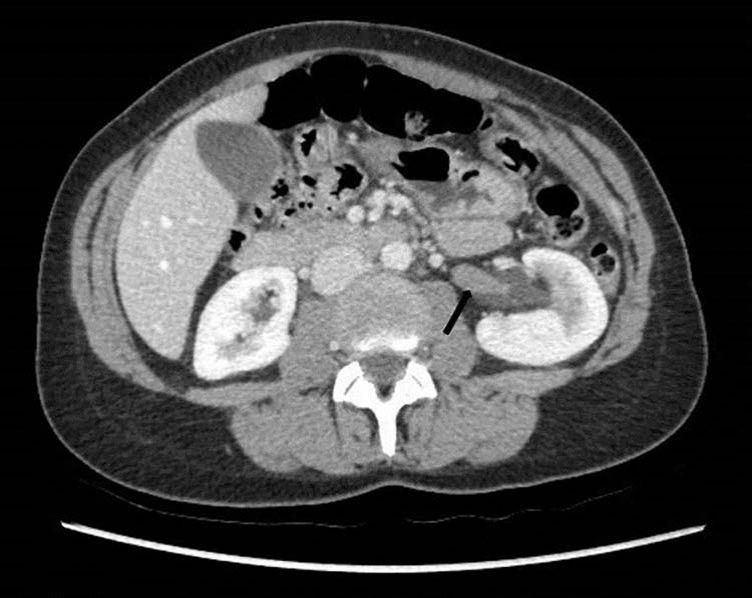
Computed tomography on presentation showing the hypodense filling defect extending from the left renal pelvis to the proximal ureter with associated hydronephrosis (arrow).
